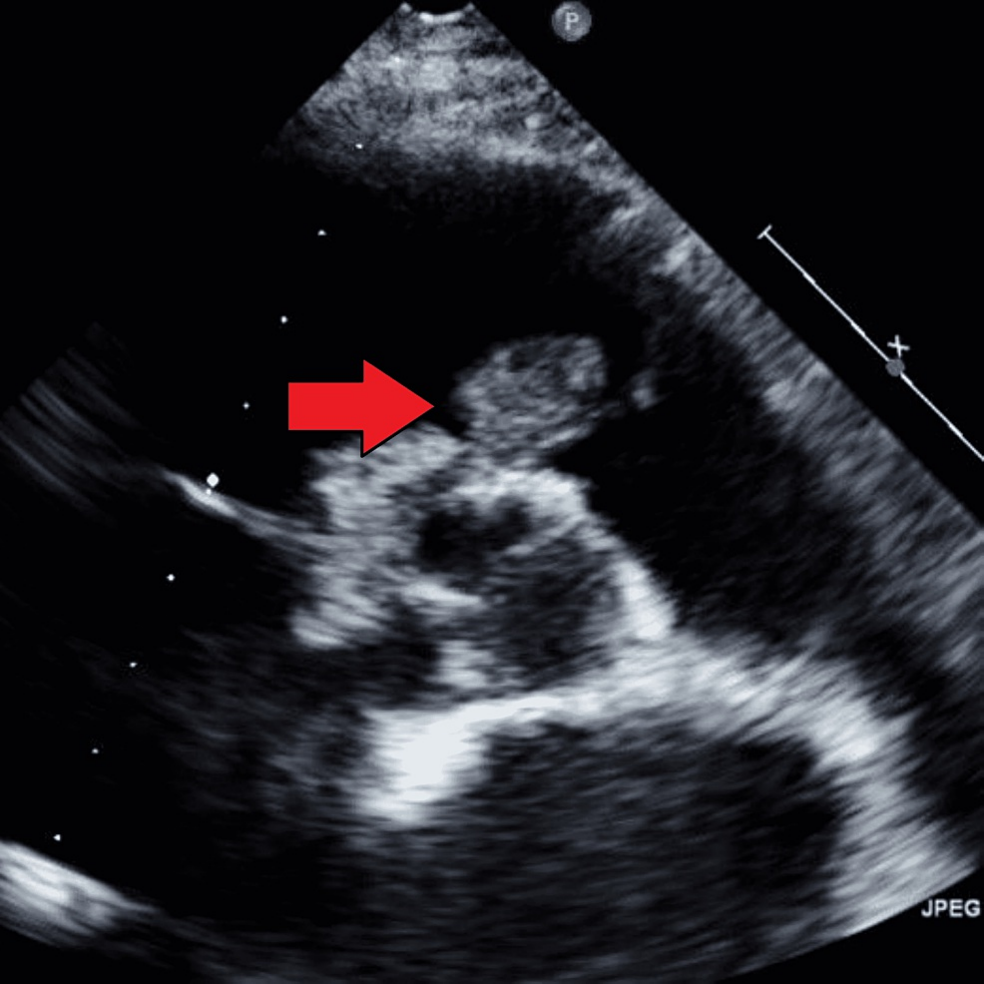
A pedunculated mass attached to the pulmonic valve is seen on a transthoracic echocardiogram.
